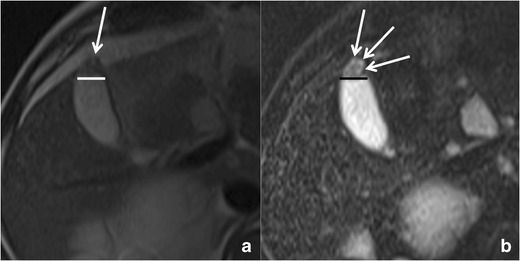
Gallbladder adenomyomatosis: typical MRI findings. On MRI, GA can be identified as a mural thickening (*line*) containing small T2-hyperintense spaces representing RAS (*arrows*). RAS can be better identified on fat-saturated T2-weighted images (b) than on non-fat-saturated ones (a)
